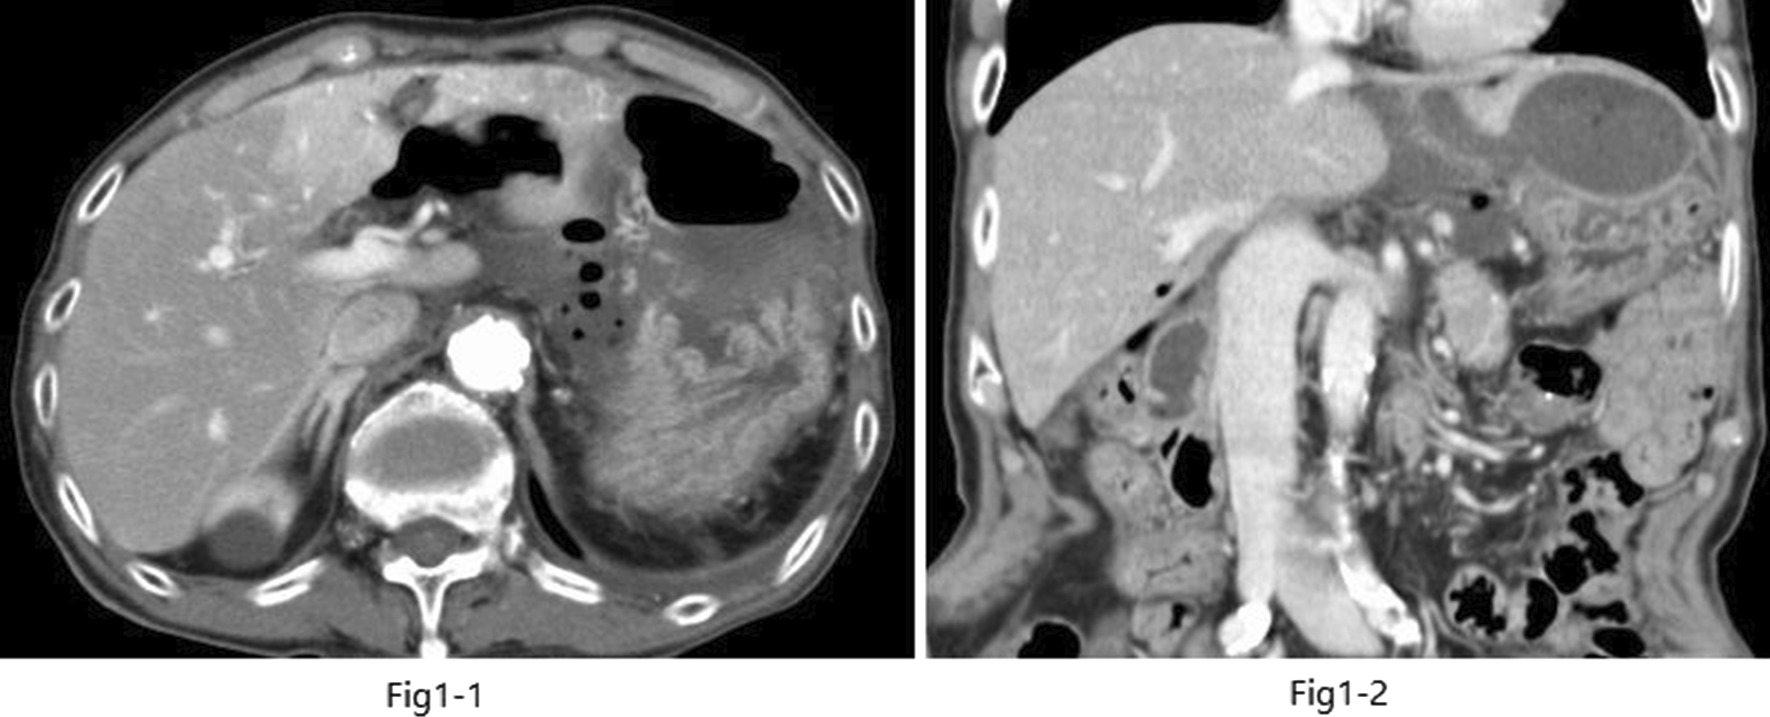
(1) Computed tomography scan (coronal view); an abscess is visible pressing on the stomach. (2) Computed tomography scan (axial view of the same location as in 1)
